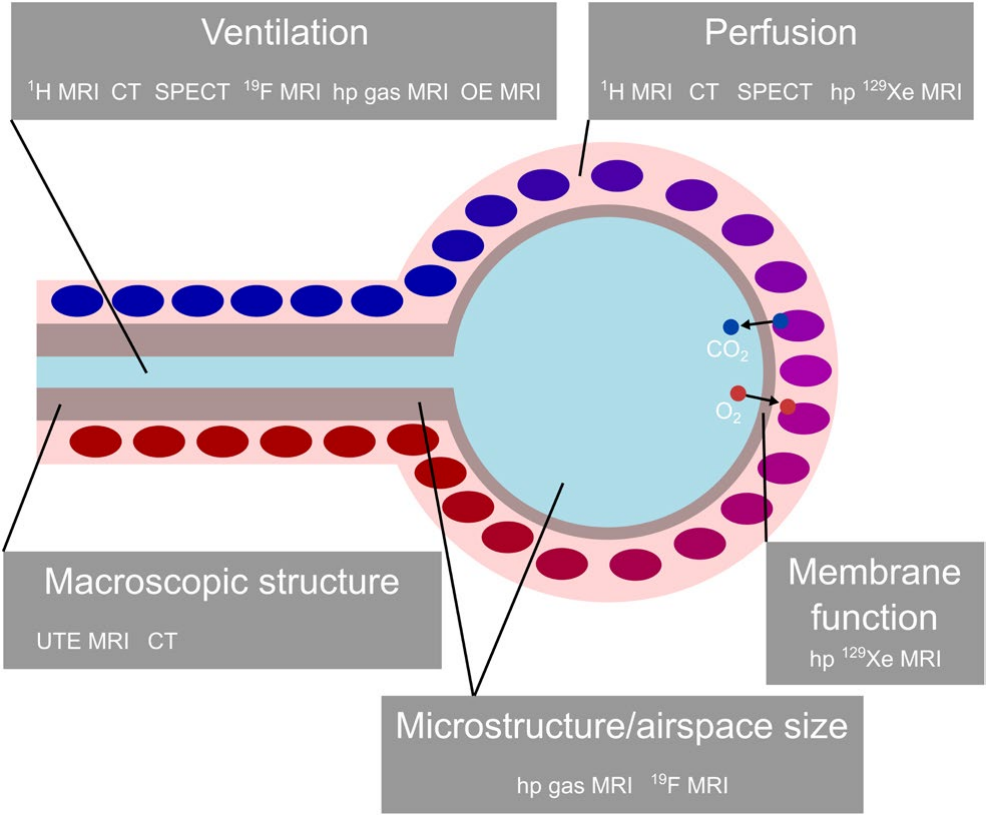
Overview of the methods available for functional lung imaging and described in this article. The methods for assessment of structure/membrane function are roughly sorted according to the length scales probed. CT, computed tomography; hp, hyperpolarized; OE, oxygen‐enhanced; SPECT, single‐photon emission computed tomography; UTE, ultra‐short echo time.
